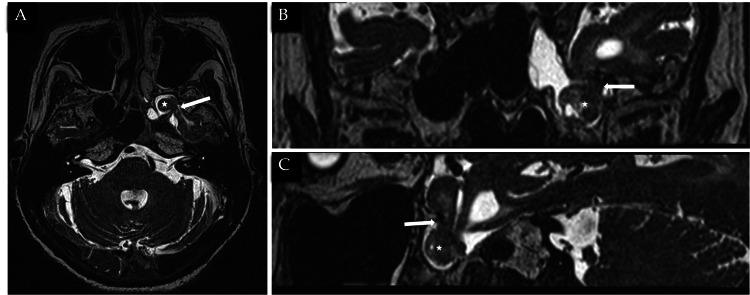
MRI T2 sequences in the axial (5A), coronal (5B), and sagittal (5C), showing brain parenchymal herniating through the defect along the posterolateral sphenoid sinus wall (white arrow) and resultant encephalocele (white star).
